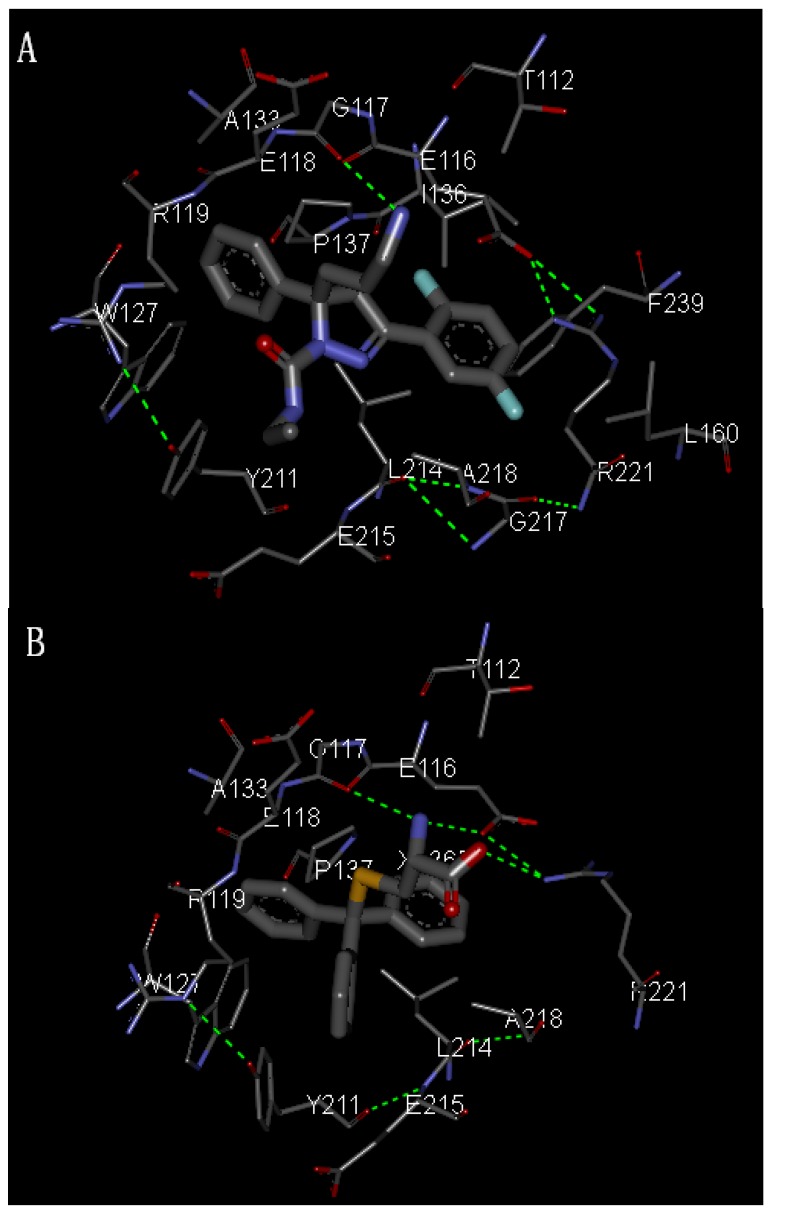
(A) Eg5 X-ray structure of the allosteric binding site with the B_12_ inhibitors in the binding site (PDB: 2G1Q); (B) Stereo plot showing STLC in the binding site (PDB: 2WOG).
